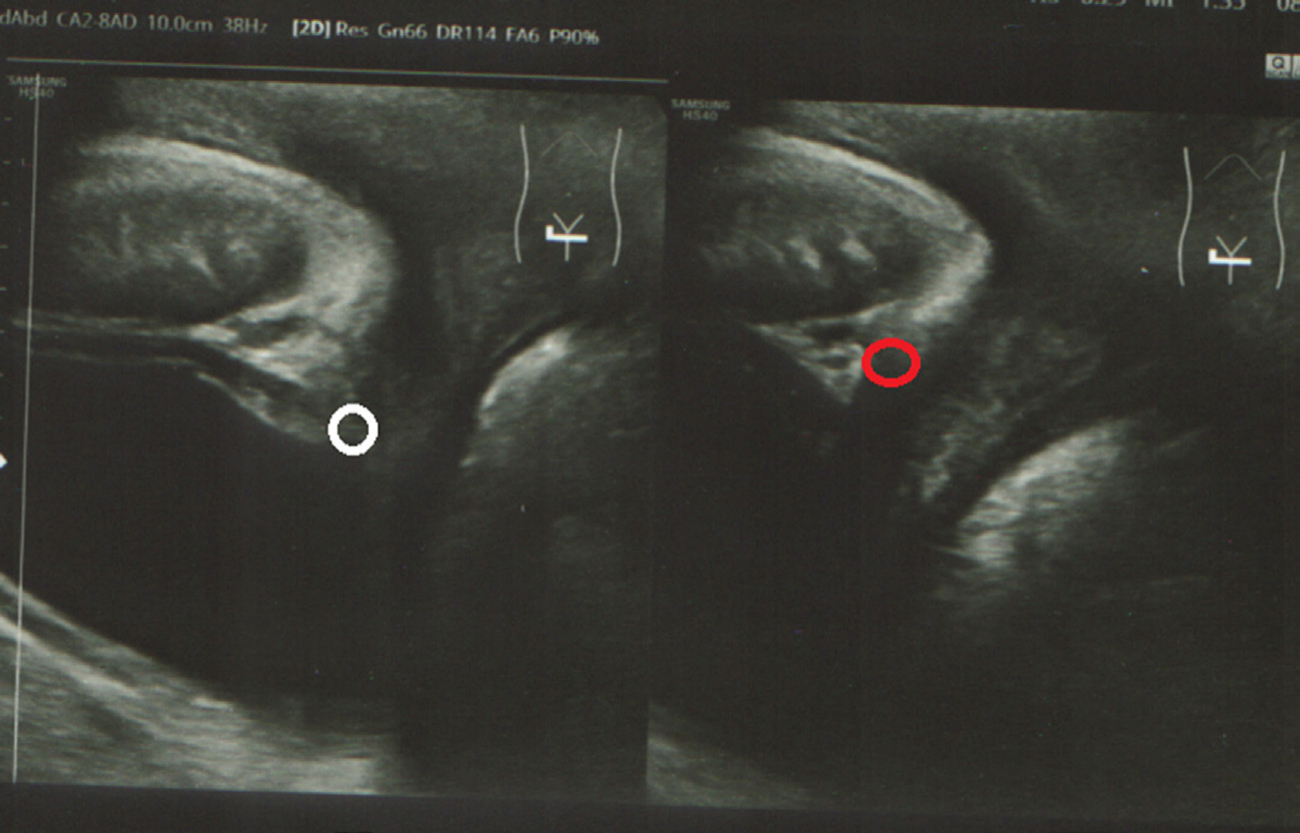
Perineal ultrasound: bladder neck hypermobility (circles).
